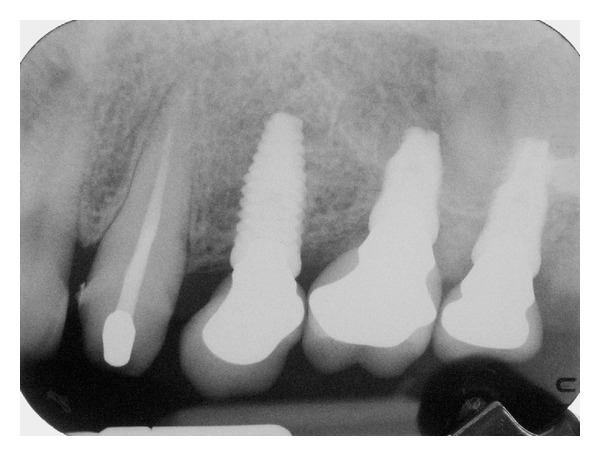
Radiographs taken at 6-month followup.
